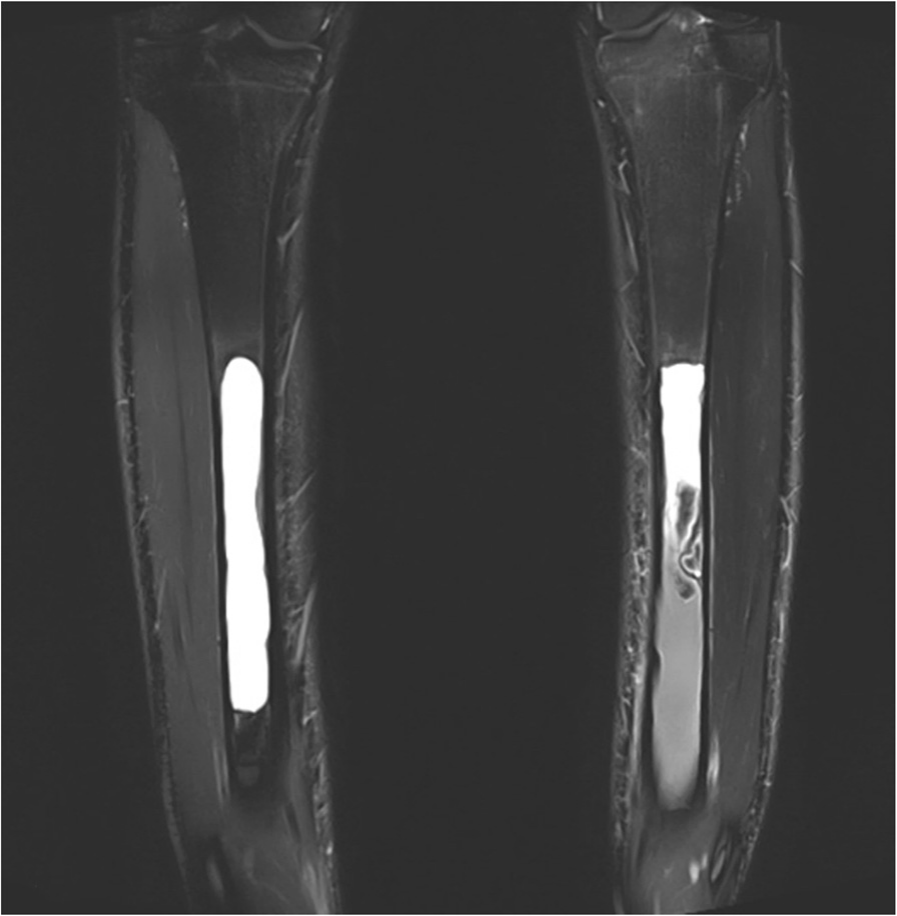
T2-weighted coronar MRI showing extensive bilateral diaphyseal cyst-like bone lesions
